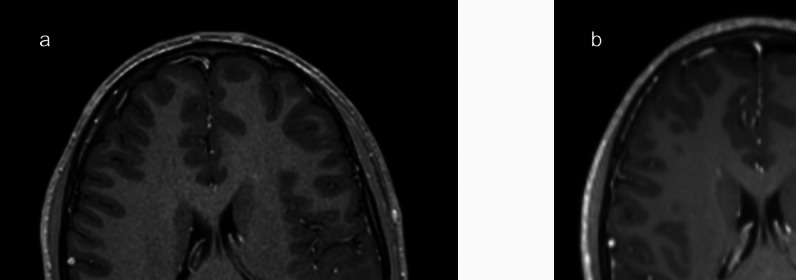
Case 4 – Pre-operative MRI (axial view) demonstrated an enhancing tumour in the left superior temporal gyrus (a). Post-operative imaging confirmed a complete resection (b)
